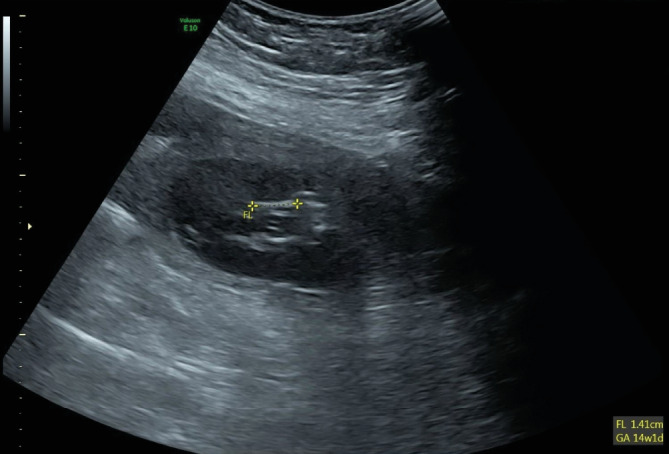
Ultrasound shows the femur length (FL) measuring 1.41 cm which equals 14 weeks and 1 day.
